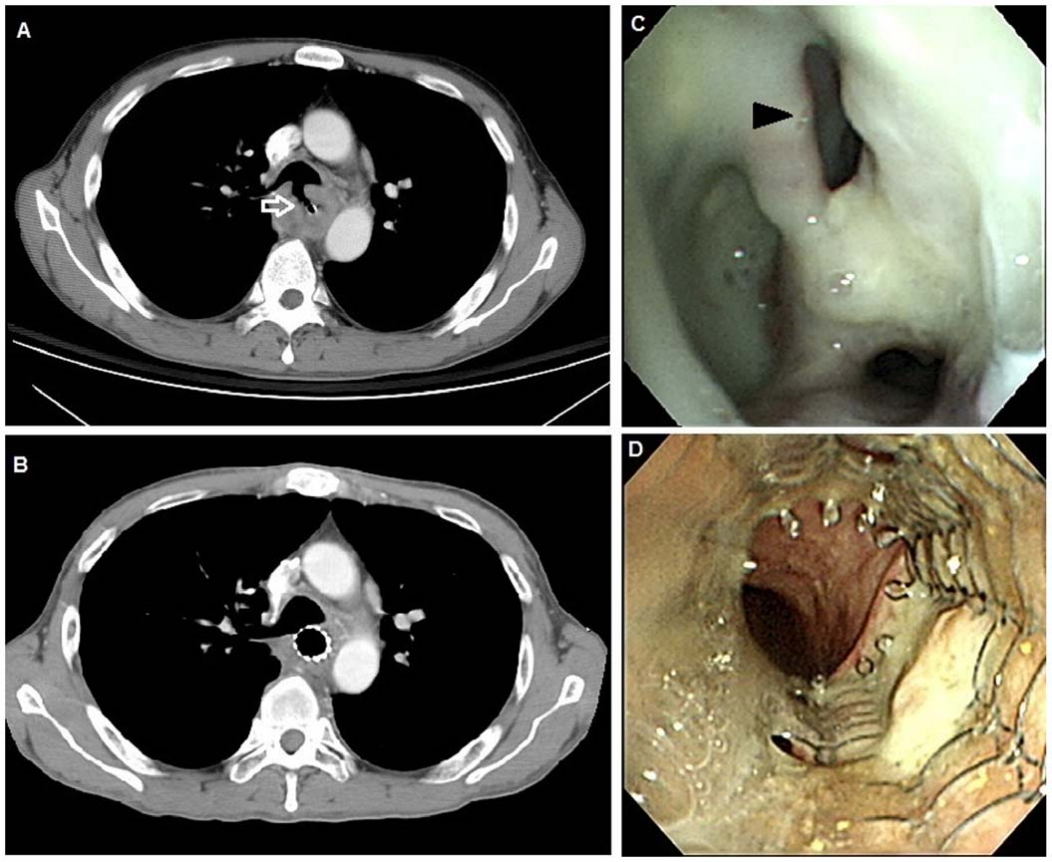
Representative images before and after esophageal metallic stent placement. A. Computed tomography of the chest obtained before stent placement showed a tracheoesophageal fistula (arrow). B. Computed tomography of the chest obtained after stent placement showed a metallic stent in the esophagus covering the tracheoesophageal fistula. C. Before stent placement, endoscopic picture showed a protruding mass with a hole in the esophagus, suggesting esophageal cancer with a tracheoesophageal fistula (arrowhead). D. Endoscopic picture of an esophageal metallic stent in place one month after insertion.
